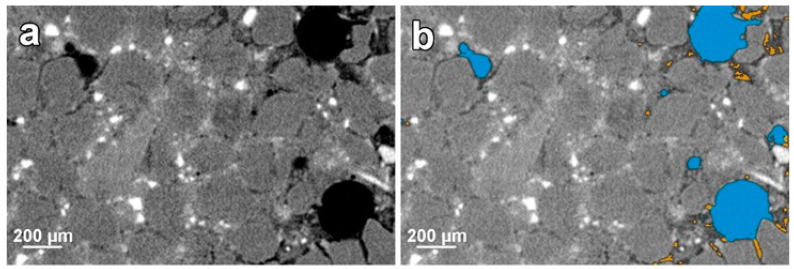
An example of an axial view of μ-CT slices inside the VOI (a) with voids indicated by black colour and (b) segmented entrapped-air voids (highlighted in blue colour) and formed microcracks (highlighted in orange colour) of a sample composed of fine-grained cement (FGC) aged for 4 weeks.
